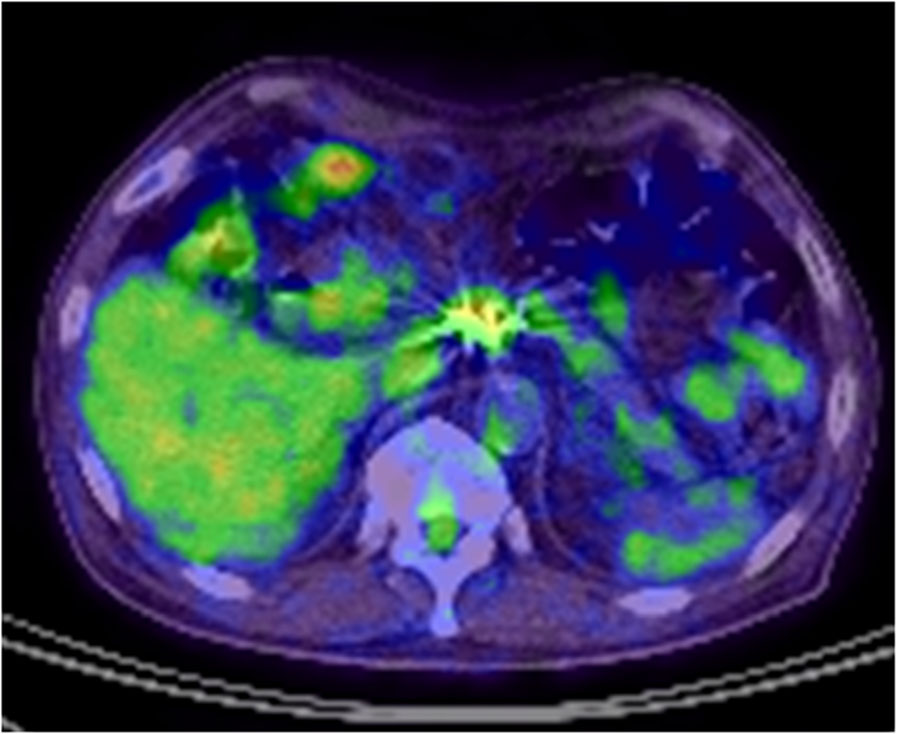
FDG-PET after three subsequent courses of GS. The main tumor of the pancreatic body had an abnormal accumulation of SUV max 3.8. Distant metastasis was not detected
